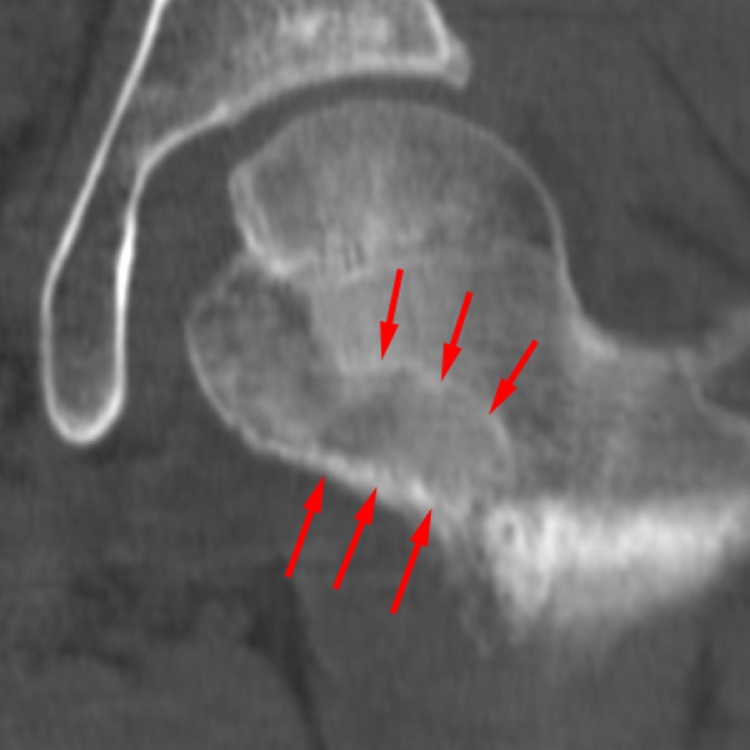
A reconstructed CT image at two weeks post-surgery. Red arrows show the route taken to reach the tumor.
